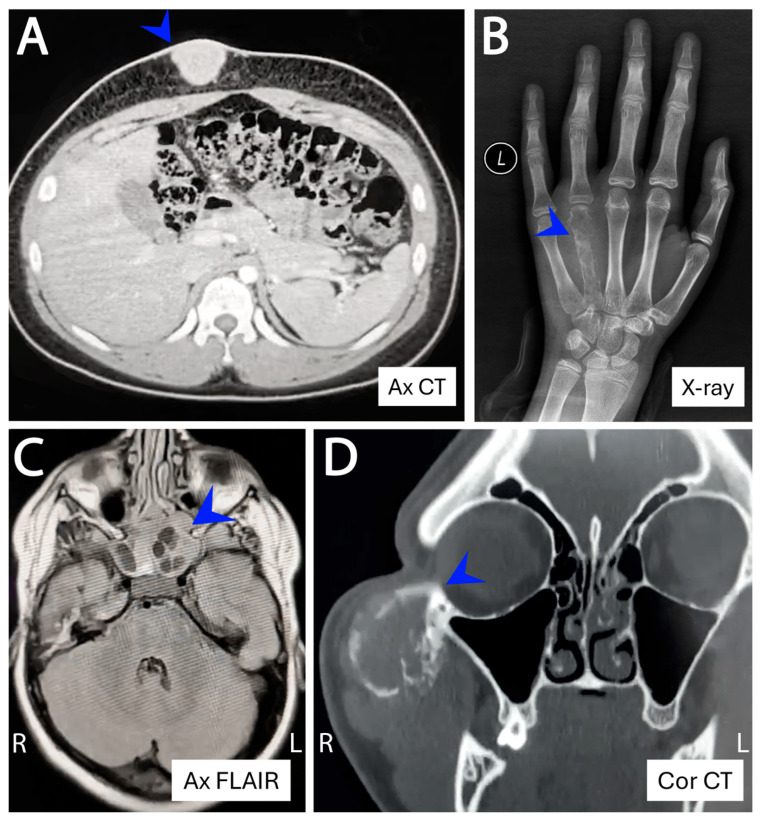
Ewing sarcomas at unusual locations. (A) Subcutaneous lesion at the epigastric region in a 14-year-old male. (B) Skeletal tumor of the 4th metacarpal bone in a 12-year-old female. (C) Head and neck lesion at the sphenoid–ethmoid sinuses and nasopharyngeal space in a 9-year-old boy. (D) Lesion arising from the zygomatic arch in a 25-year-old man. Ax = axial; Cor = coronal; CT = computed tomography; FLAIR = fluid-attenuated inversion recovery MRI.
